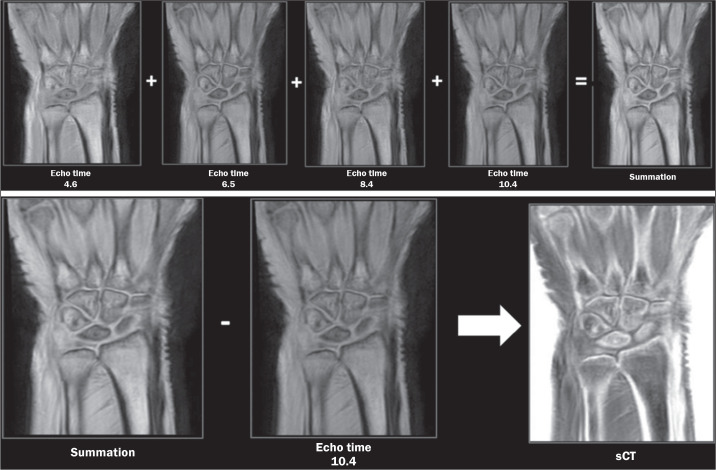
FRACTURE sequence MRI acquisition technique in which different echo times
